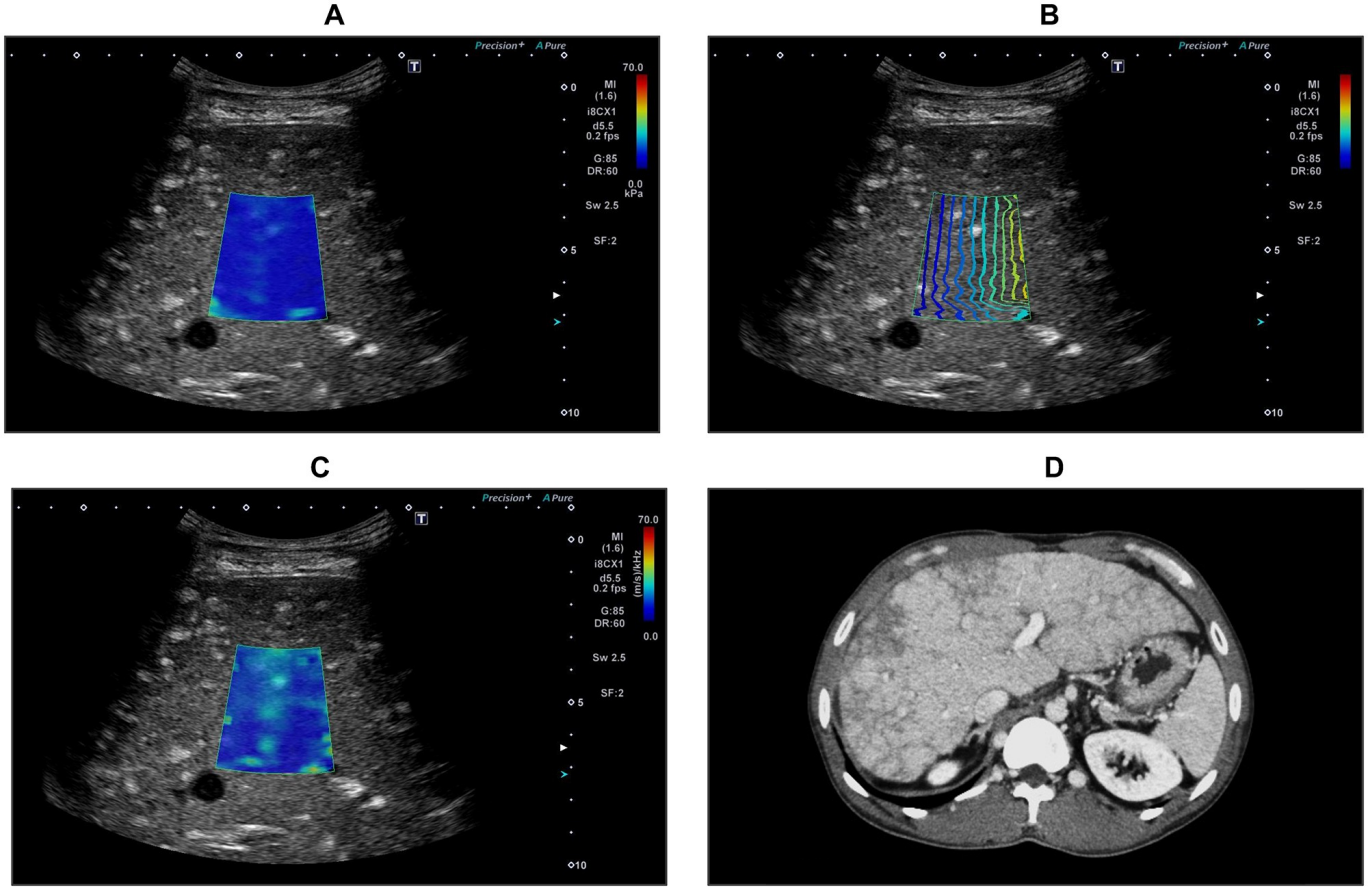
Two-dimensional shear wave elastography (2D-SWE), shear wave dispersion (SWD), and abdominal computed tomography (CT) images. 2D-SWE color map (a), propagation map (b), SWD color map (c), and abdominal CT (d) in patients with FALD.
